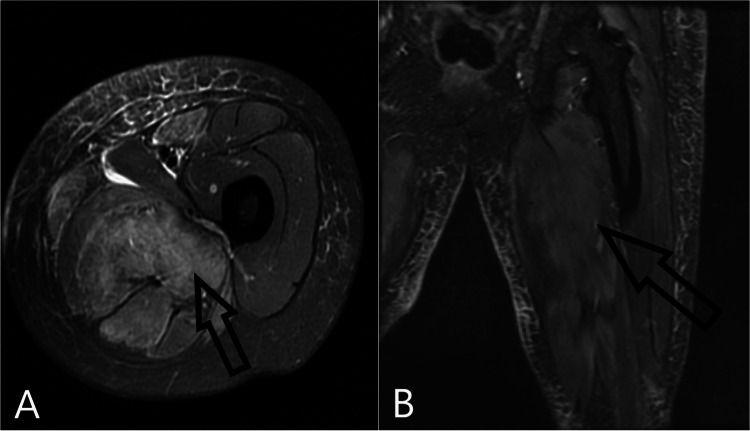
MRI of the left thigh. (A) Axial plane and (B) Coronal plane. Arrows highlight diffuse edema in left thigh muscles in different MRI planes.
